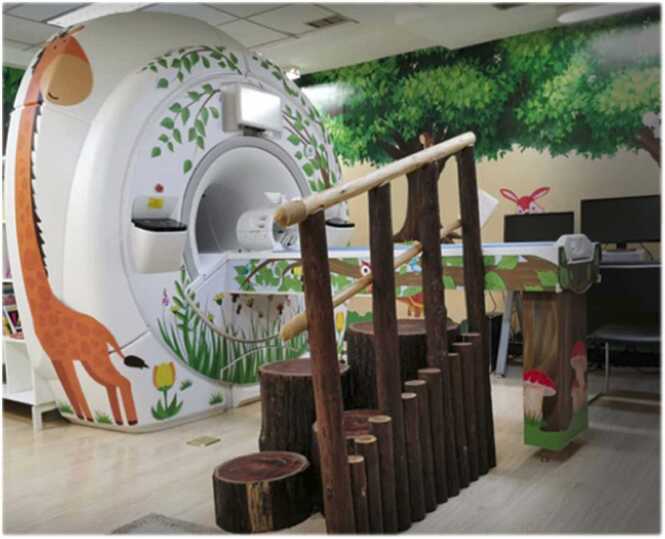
The mock scanning facility in the Magnetic Resonance Imaging Research Center at IPCAS.
